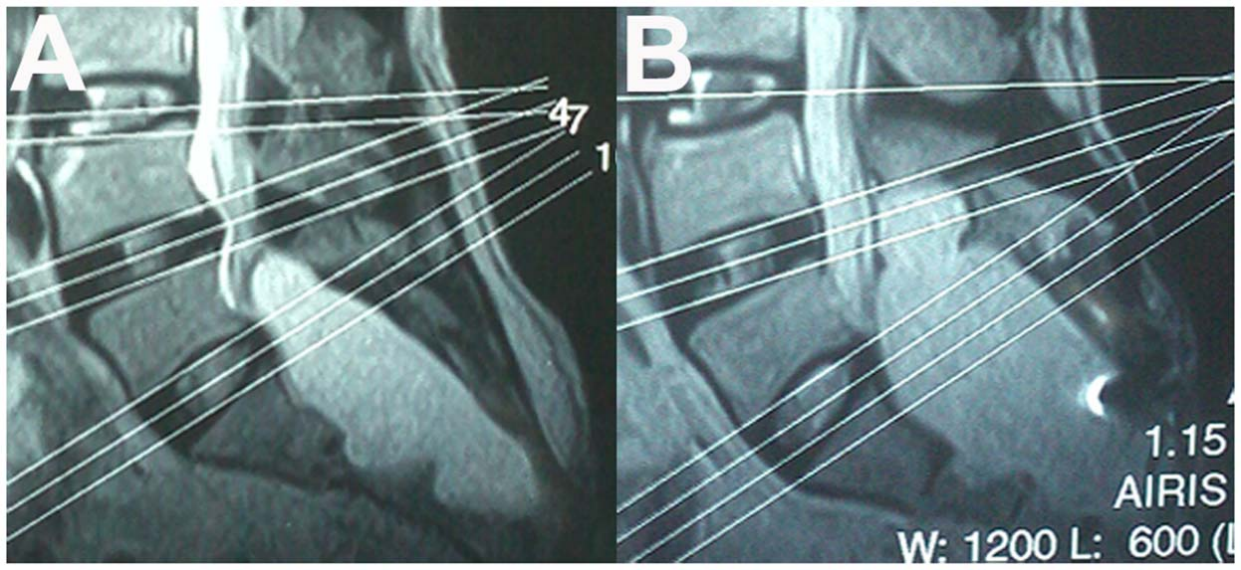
MRI study of recurrence of the cyst.A: Preoperative MRI showing a cyst in sacral spinal canal. B: Postoperative MRI showing the cyst reoccurring 8 months after the operation.
